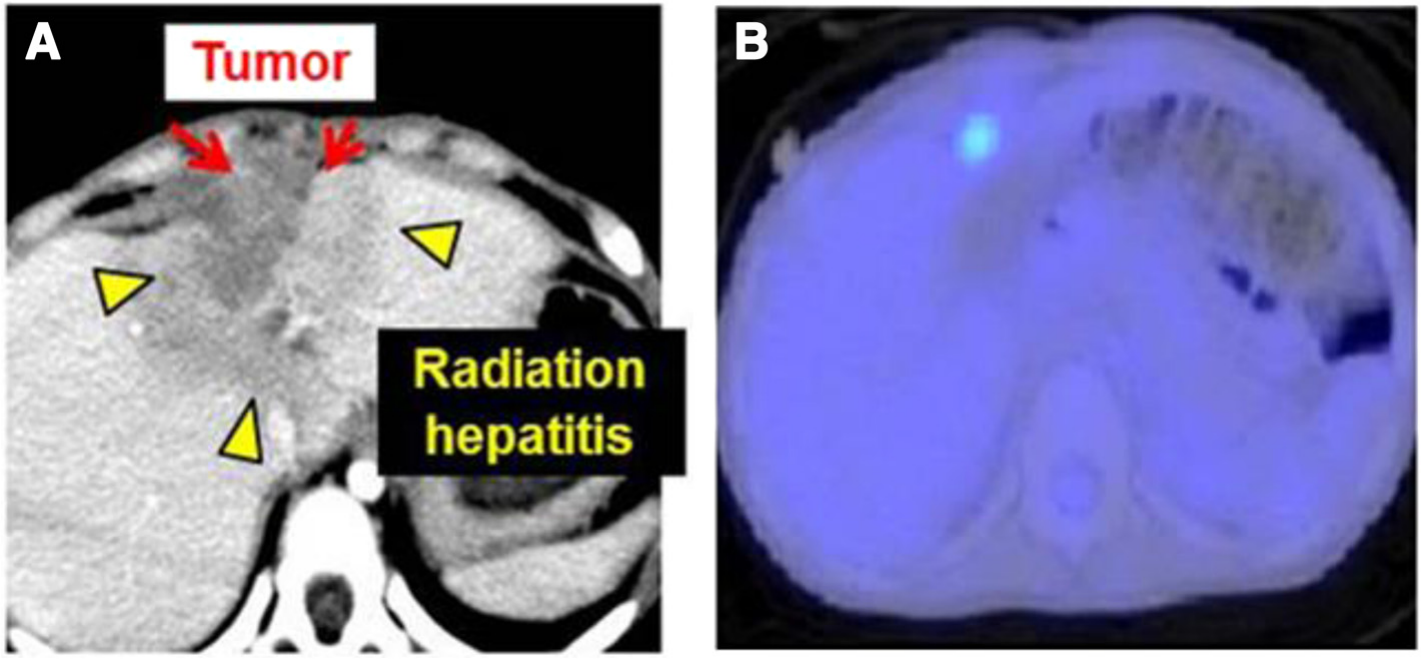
These images were contrast enhanced CT image a and PET-CT image b, at 1 year after PBT. In figure a, local recurrence of the tumor is enhanced at the edge of irradiation, and FDG accumulated same place
